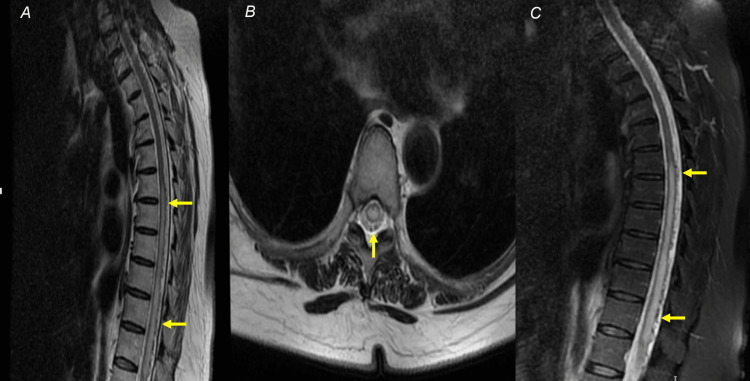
Spine magnetic resonance imaging. (A) Enlarged perimedullary vessels in the posterior epidural region in the T2 sequence (yellow arrows); (B) T2 sequences in the axial section occupy 80% of the cross-sectional area of the spinal cord with hypointense signal voids (yellow arrow; (C) STIR sequence with hyperintense signal image from T6 to T11 levels in the spinal cord caused by cytotoxic edema or myelomalacia (yellow arrows). STIR: short tau inversion recovery
